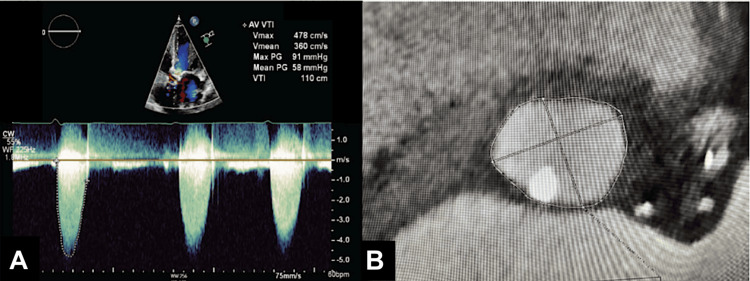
(A) Transthoracic echo shows severe aortic stenosis. (B) Prominent calcific nodule extending into the annulus and left ventricular outflow tract (LVOT) with aortic annular area measured at 418 mm². Figures reprinted from [12], ©2025 HMP Global. Used with permission.
